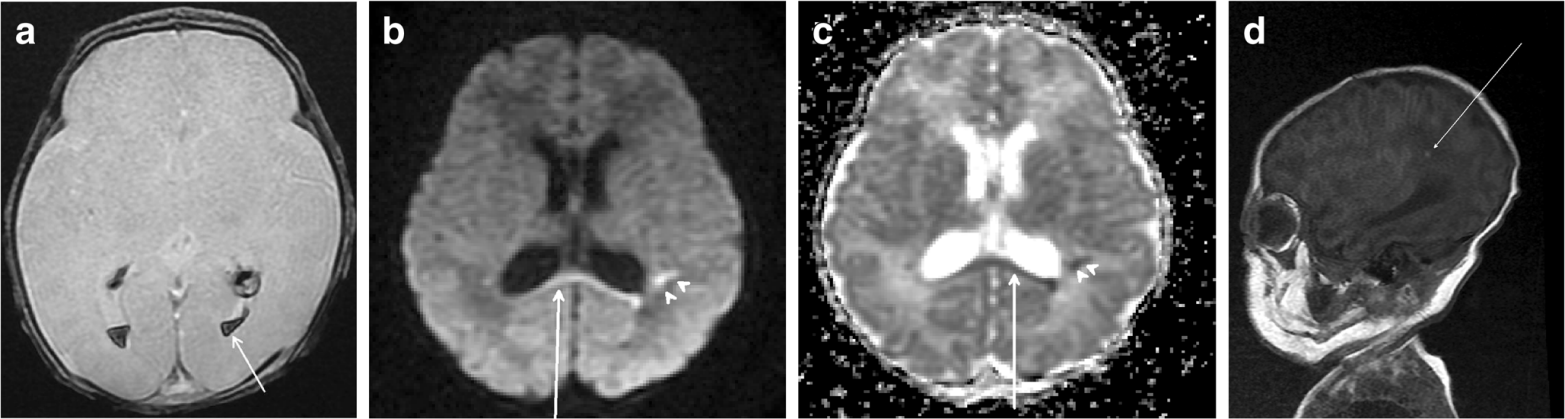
A 13-day-old female neonate was transferred to our hospital because of a seizure. She was delivered by an elective cesarean section and had a PDA and ASD. Apgar scores at 1 and 5 min were 8 and 10, respectively. MRI obtained 16 days postnatally showed a bilateral intraventricular hemorrhage on axial GE imaging and b, c diffusion restriction of the corpus callosum (arrow) and high-signal foci in both frontoparietal periventricular white matters (arrowheads) on axial DW and ADC map. d Several high-signal foci were observed in the left periventricular white matter on T1 sagittal scan (arrow). Bayley scale evaluation at 8 months showed normal development
